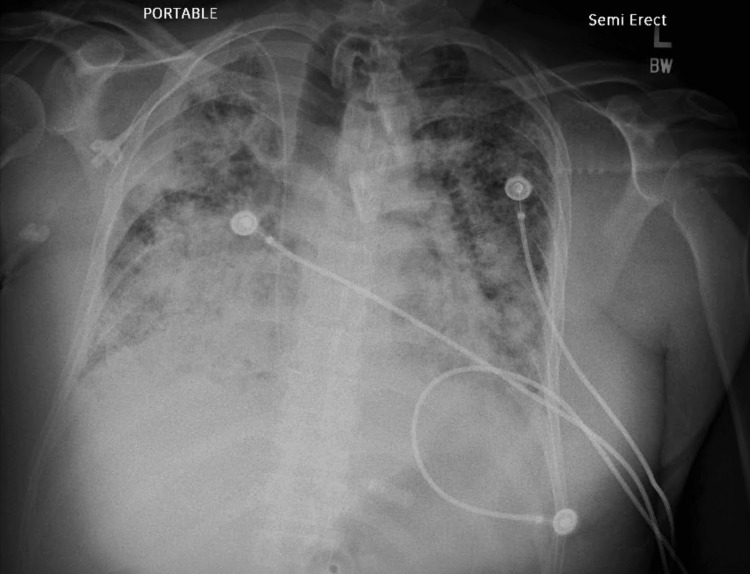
Patient’s X-ray at day 61
